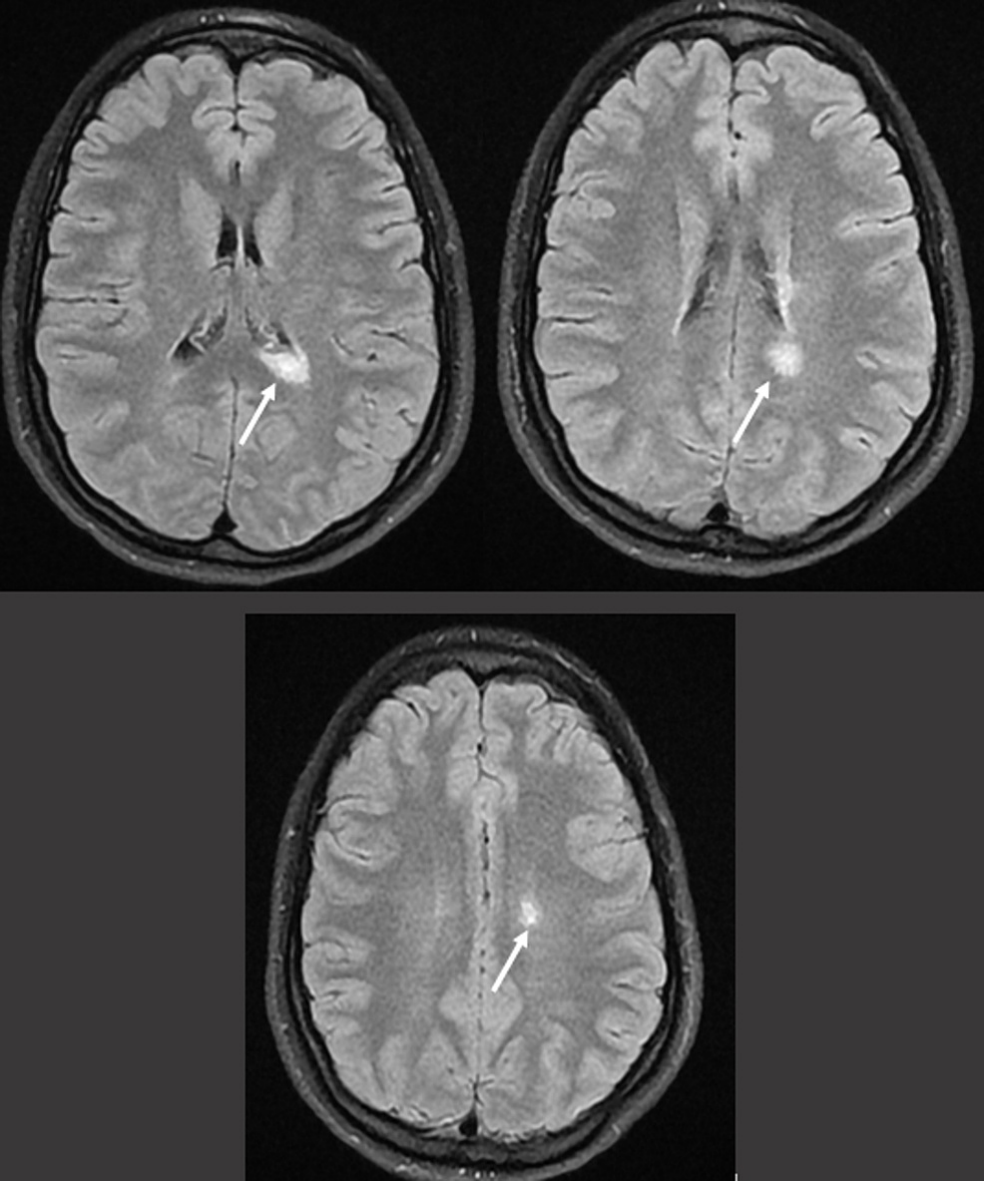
Brain MRI follow-up at six months showing three new hyperintense FLAIR periventricular lesions (arrows) MRI: magnetic resonance imaging; FLAIR: fluid-attenuated inversion recovery
